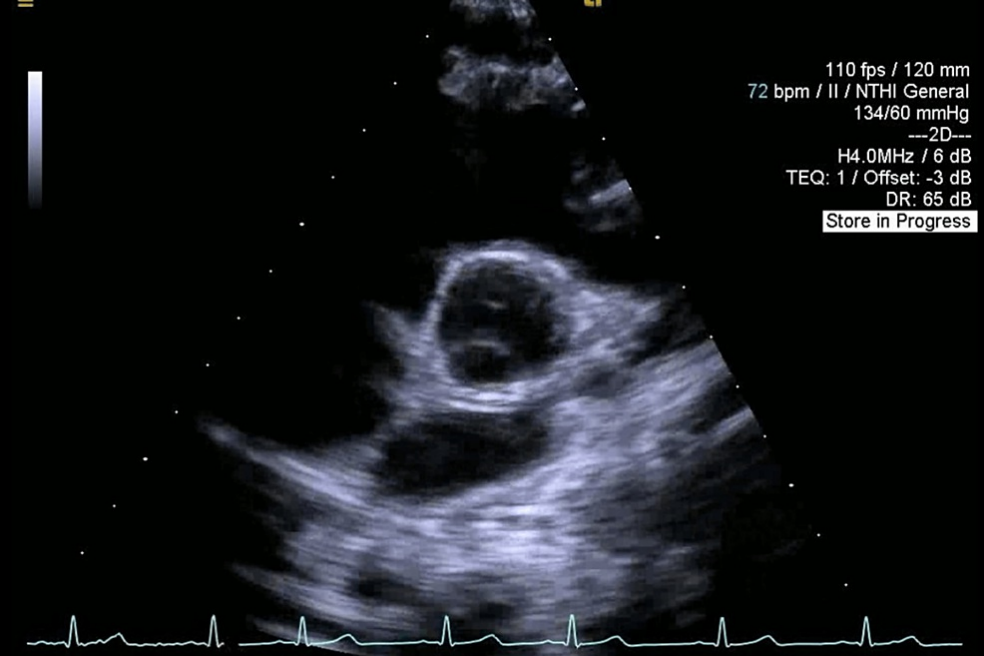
Transthoracic echocardiogram, parasternal short-axis aortic valve level view showing normal cardiac structure
